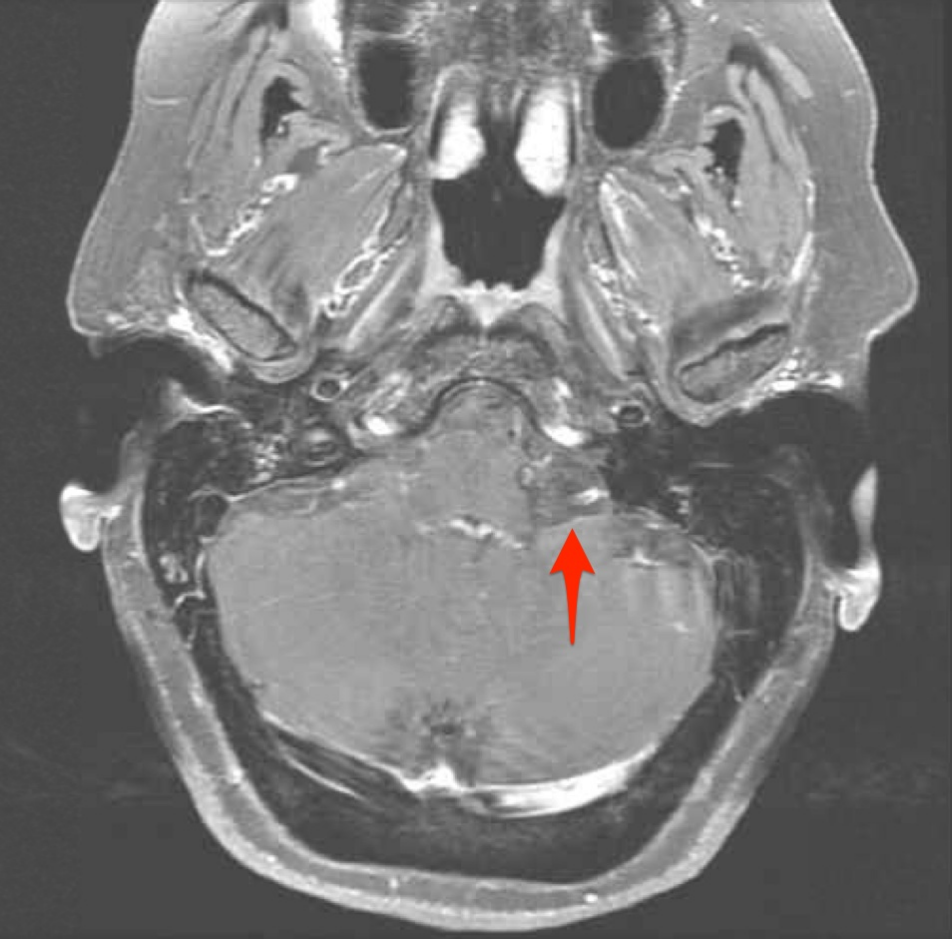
Axial T1 post-contrast fat saturation sequence magnetic resonance imaging (MRI) Image demonstrates loss of signal hyperintensity within the left cerebellopontine angle mass with fat saturation techniques (see arrow), as is typical of fatty lesions.
